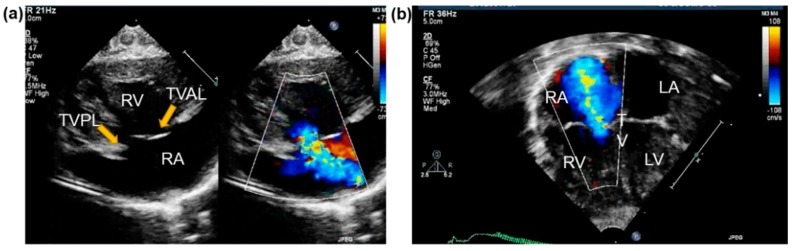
Visualization of severe tricuspid reguritation using two different 2DTTE views and color flow Doppler: (a) a parasternal long-axis inflow view, and (b) an apical four-chamber view of a newborn with a severe pulmonary hypertension due to diaphragmatic hernia. Images courtesy of Dr. Mir and Dr. Burkhart from the Children’s Heart Center at the University of Oklahoma Health Sciences Center (OUHSC).
